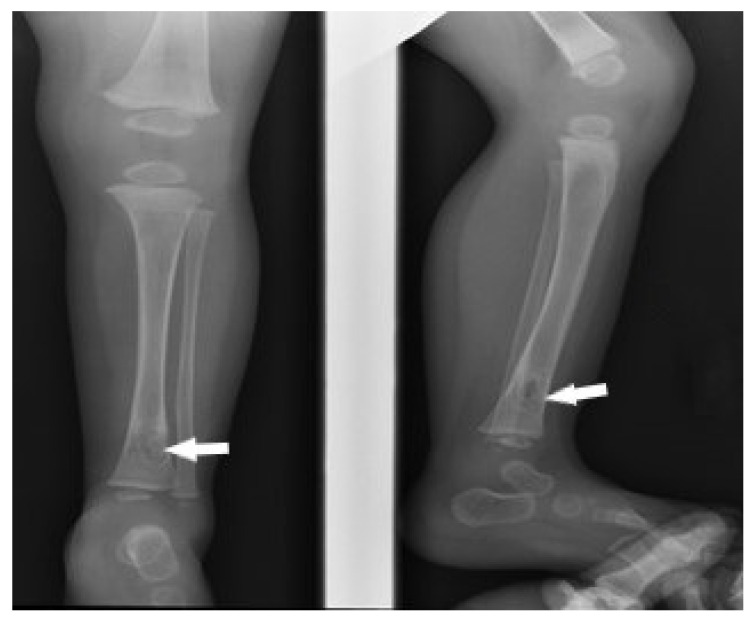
Radiographic image of changes in the distal metaphysis of the tibia (white arrows)—after 3 months of treatment. Partial but significant regression of bone lesions.
